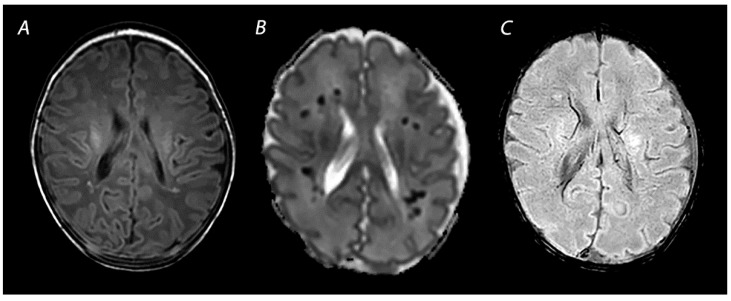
MRI performed on day 7 showing punctate white matter lesions. While these lesions are subtle on T1 weighted imaging (A), the abnormalities are much clearer and more numerous on the ADC map (B). Susceptibility weighted imaging can be used to rule out hemorrhagic abnormalities (C).
